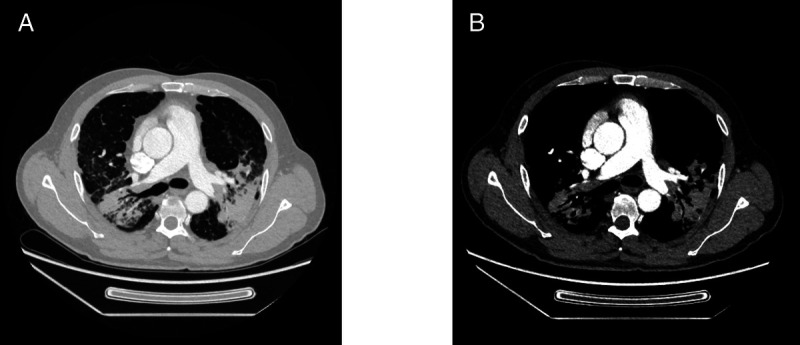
Chest CT findings. The chest CT of the patient reveals diffuse ground-glass opacities in both lungs, and multiple mediastinal lymphadenopathies. (A) CT scan in lung window. (B) CT scan in mediastinal window. CT: Computed tomography.
